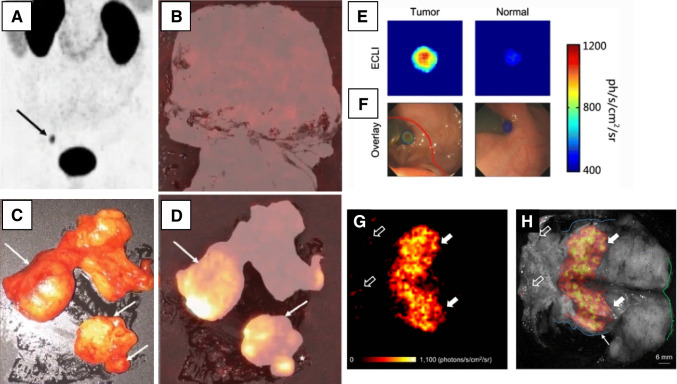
Intraoperative use of Cerenkov luminescence imaging. Cerenkov luminescence imaging for oligometastatic prostate cancer after [^68^ Ga]Ga-PSMA-11 PSMA-PET/CT (A) indicating prostate tumor close to the surface of the organ. Example of Cerenkov imaging detecting no tumor close to the surface of the resected prostate (B). Macroscopic lymph node metastasis and two different nodes were palpable (C, arrows). Corresponding Cerenkov imaging detecting PSMA-positive lymph nodes (D) [70]. Endoscopic Cerenkov luminescence imaging, after injection of 2-[^18^F]FDG, showing cancerous lesions of the GI tract (E, left panel) and overlaid with white-light images taken by clinical colonoscopy (F) [71]. No special type invasive breast cancer Cerenkov image using 2-[.^18^F]FDG (G) and gray-scale photographic image overlaid with Cerenkov signal (H) showing increased signal from tumor is visible (white arrows) [72]
